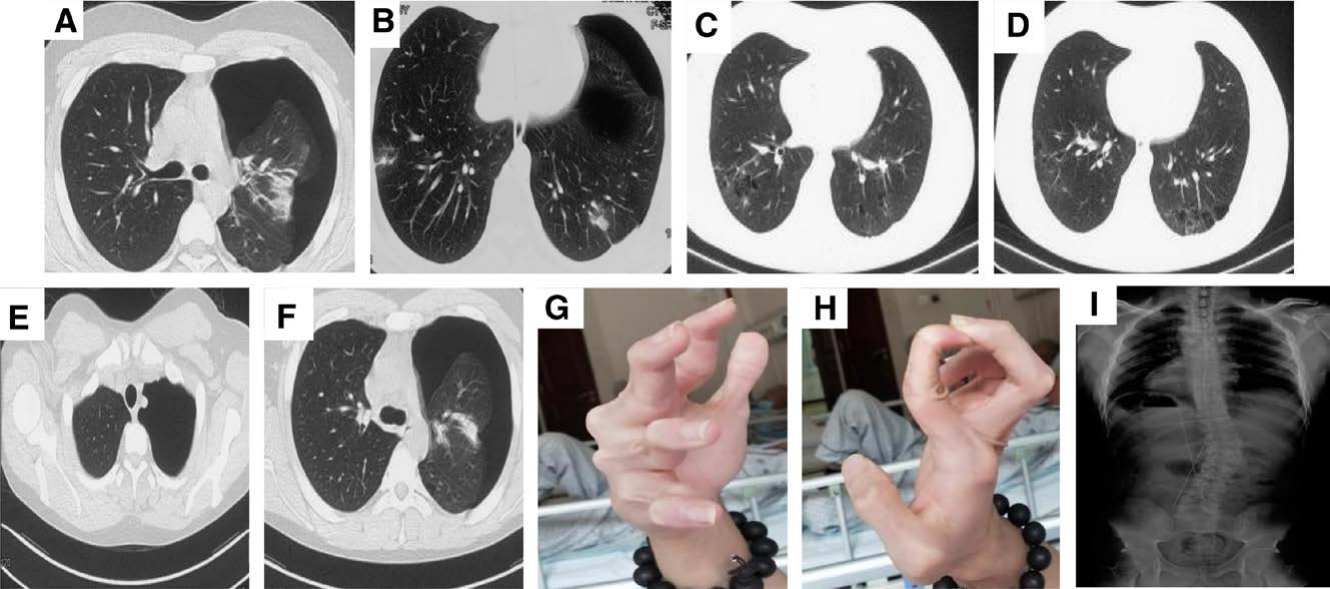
Chest CT showed left pneumothorax. Multiple pulmonary cavitary were found in the lower lobe. Nodules was observed in bilateral lower lobe (A–F). Mild hypermobility of the fingers was observed (G–H). X-ray of sacroiliac joint found his lower lumbar scoliosis was about 30° (I).
